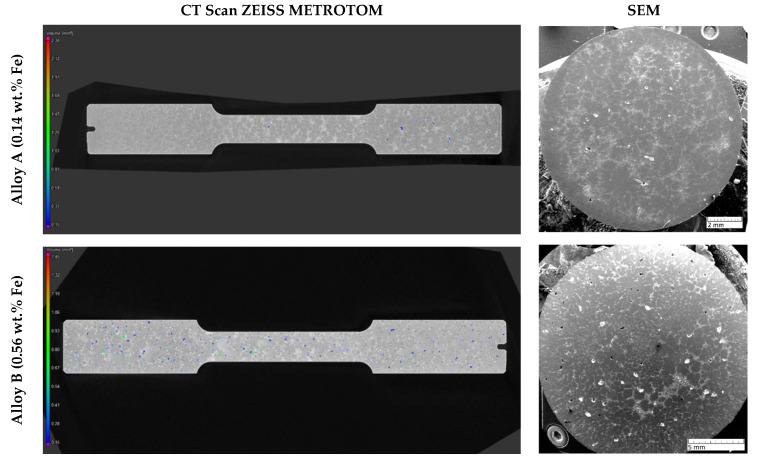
Quantification of pores using CT scan and SEM analysis, etch. 0.5% HF.
